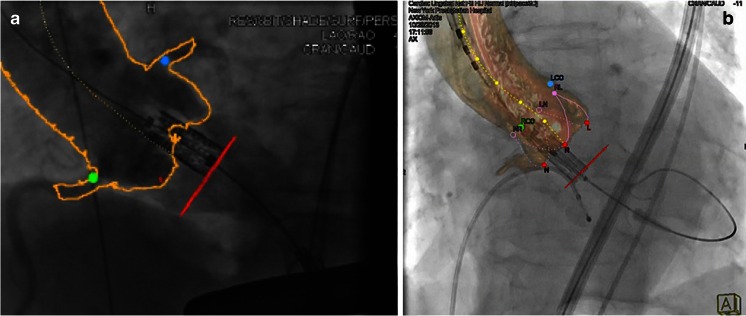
Fusion imaging during TAVR. Fusion imaging during transfemoral TAVR procedure. Both images show fusion of CT and fluoroscopy images. a The CT-gained boarders (marked *yellow line*) of the aortic root as well as the coronary ostia (*green* and *blue markers*) demonstrate perfect fusion with fluoroscopy. b A similar visualization is achieved using a semitransparent 3D model of the reconstructed aorta (based on CT data), overlaid on fluoroscopy. Both modalities enable deployment of the valvular prosthesis at the correct annular height (*red dots*). a Reprinted from [39] with permission
